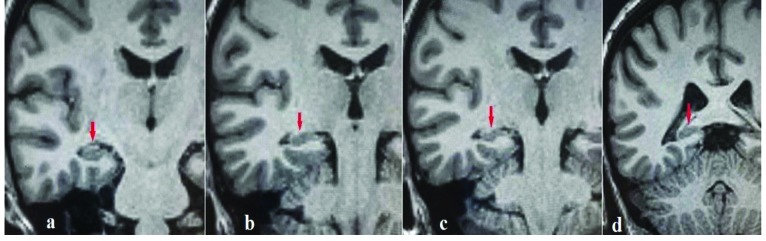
Consecutive coronal oblique T1-weighted MP-RAGE images demonstrating thehippocampal head (a), body (b, c), and tail (d) (red arrows).
